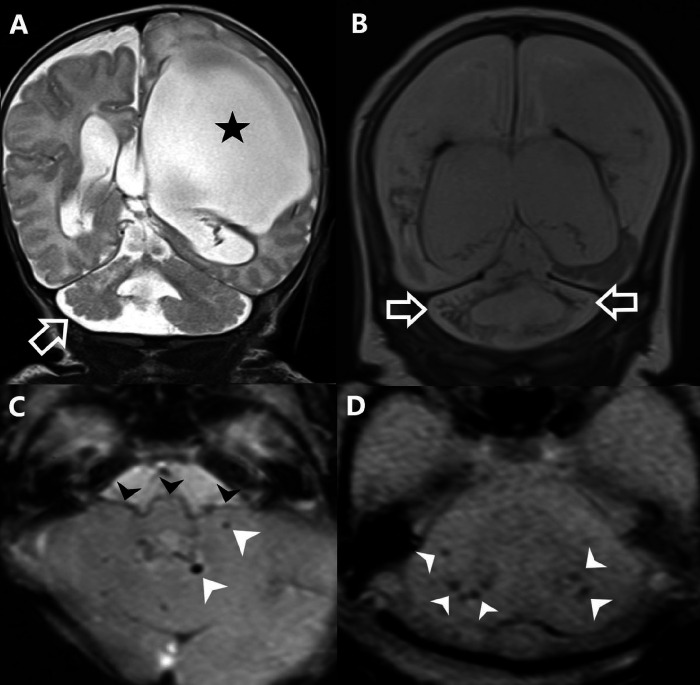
Cerebellar injury on MRI. A. Coronal T2 weighted MRI shows mild right cerebellar volume loss (arrow) contralateral to a large porencephalic cyst (star) in this patient with prior grade 4 germinal matrix hemorrhage. B. Coronal T2 MRI shows severe bilateral cerebellar volume loss (arrows) in this patient who also had a severe brain injury in the supratentorial brain. C. Axial SWI MRI shows few small hemorrhages in the left cerebellar hemisphere (white arrowheads) and superficial siderosis coating the surface of the brainstem and cerebellum (black arrowheads). D. Axial GRE shows multiple bilateral hemorrhages in the cerebellum.
